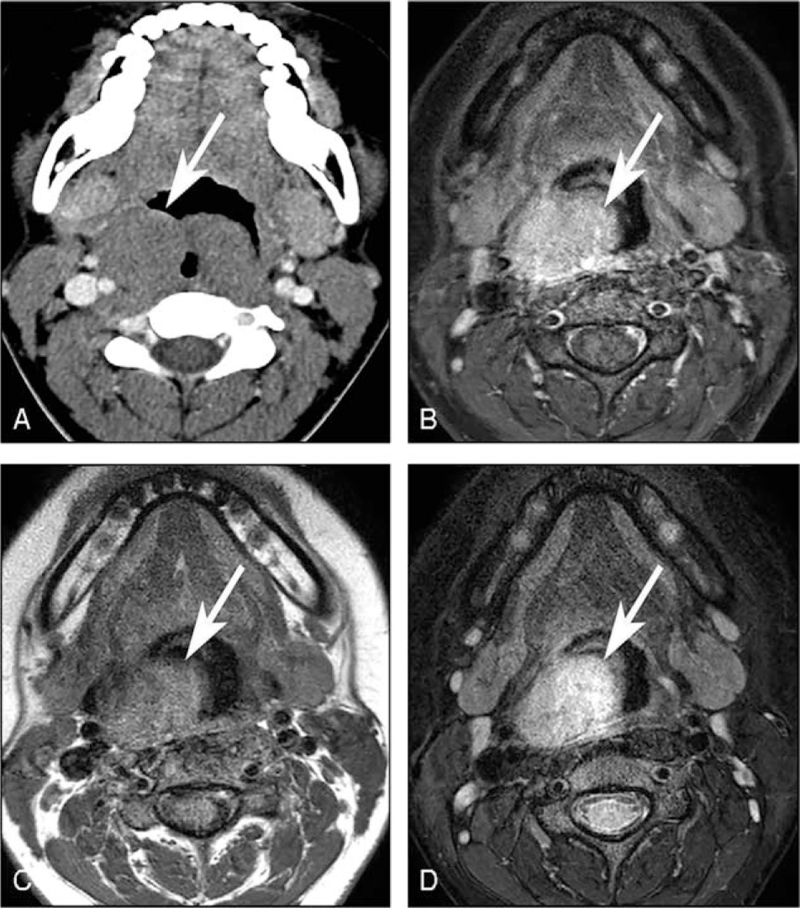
Computed tomography (CT) and conventional magnetic resonance imaging (MRI) findings. (A) CT and (B) postcontrast T1-weighted MRI images show a circumscribed solid mass with homogeneous contrast-enhancement in the oropharynx. (C) The mass shows low signal intensity on T1-weighted imaging. (D) The mass is hyperintense on fat-suppressed T2-weighted imaging. The white arrows indicate the mass.
